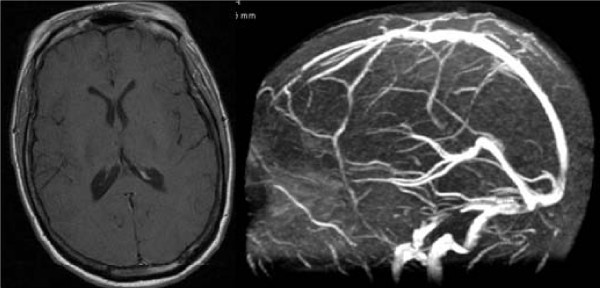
Brain MRI scan with venography. A brain MRI scan showing normal morphology, ventricular dimensions and venous drainage.
